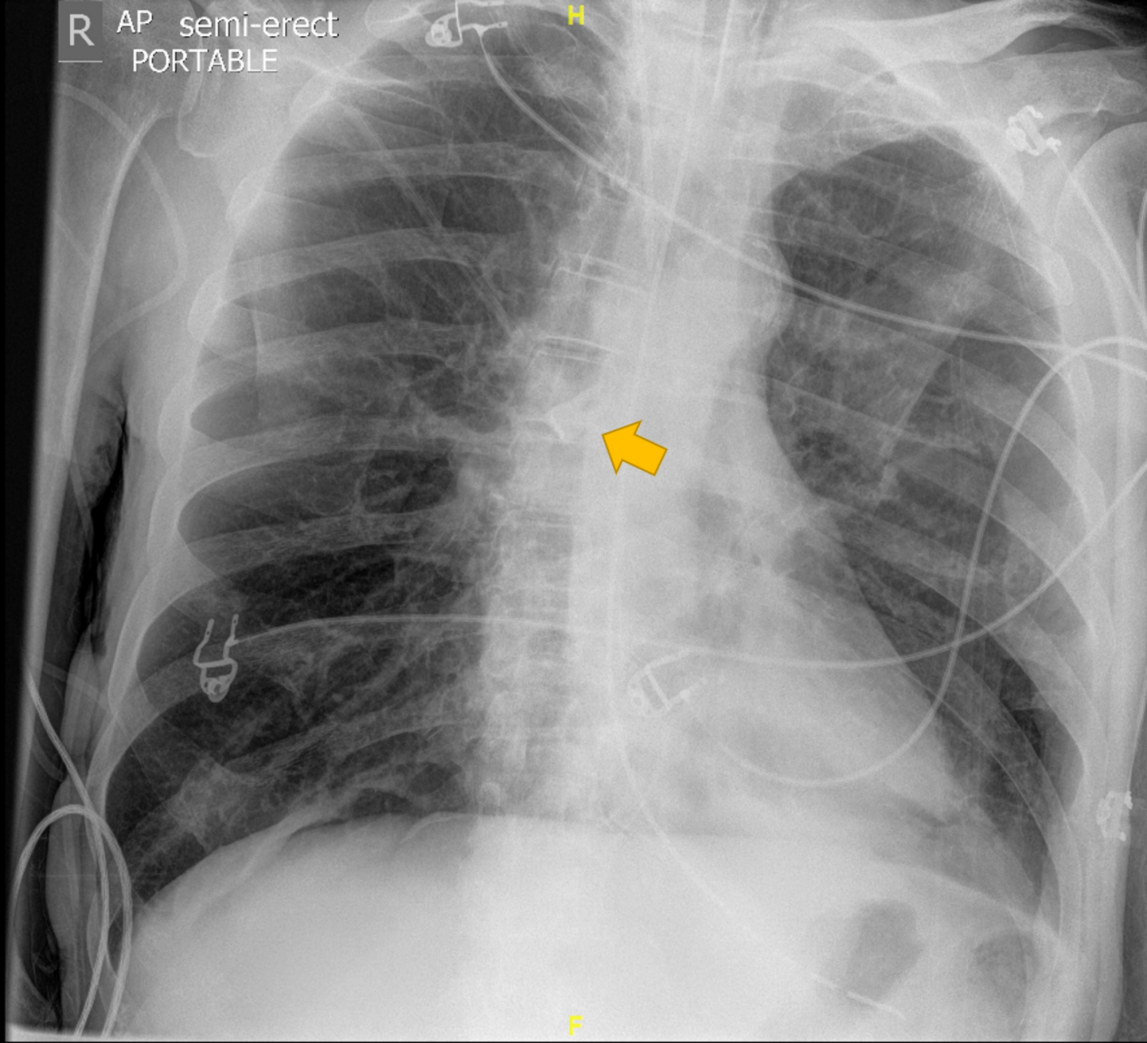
Plain radiograph demonstrating a tooth in the right main bronchus.
